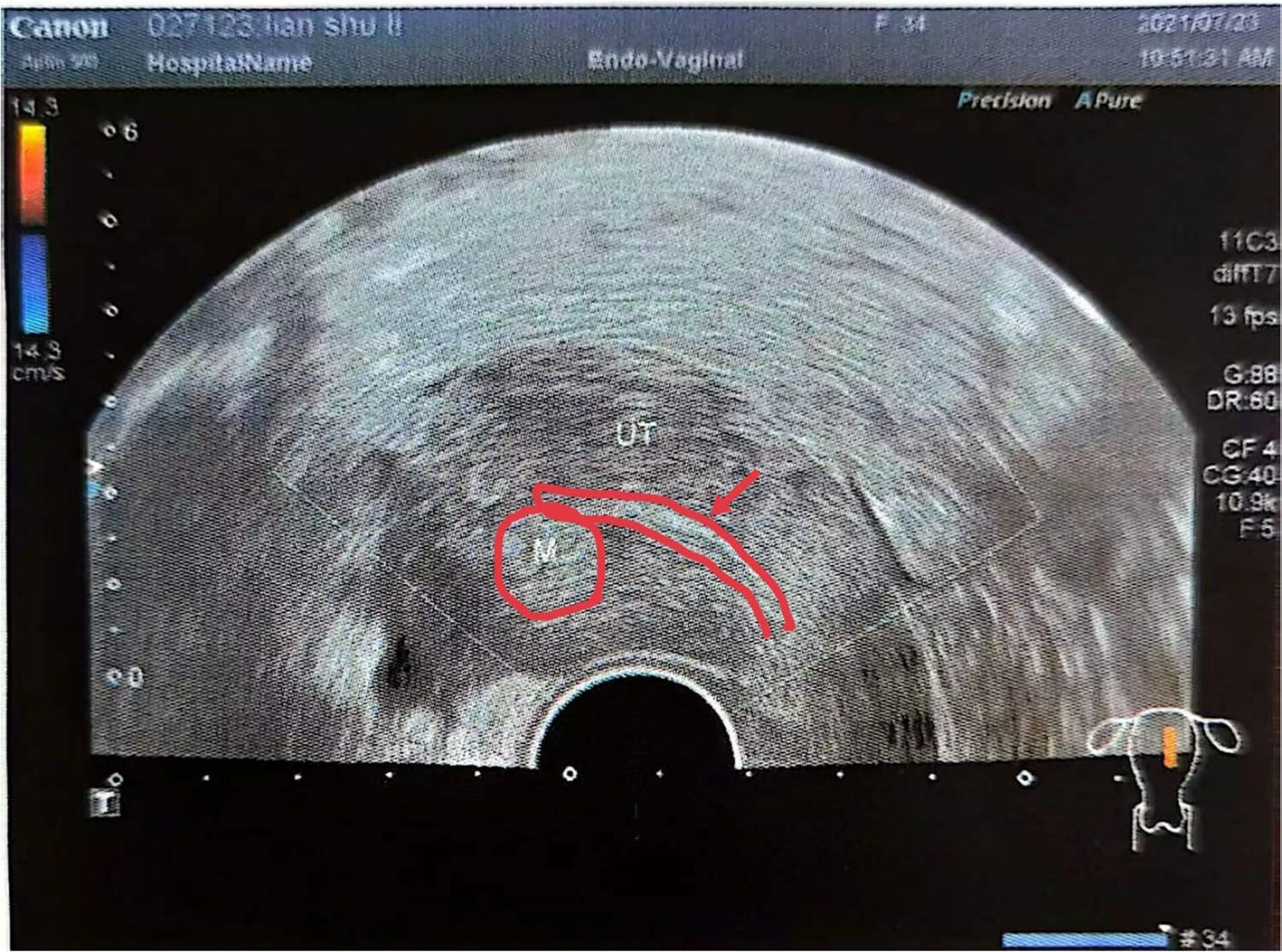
Sonographic presentation of type 3 myoma and the endometrium
